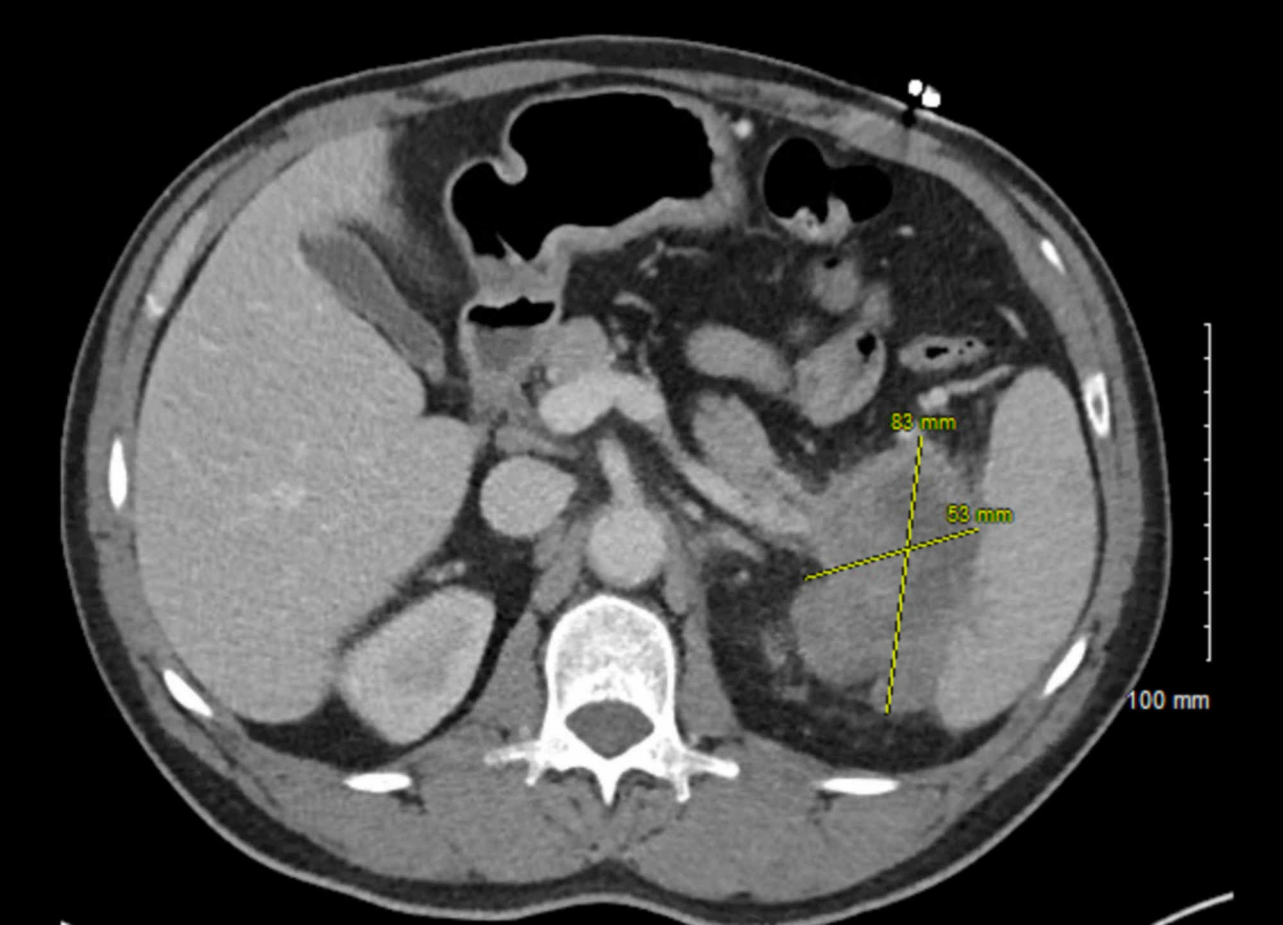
Computed tomography scan of the abdomen showing an 8.3 x 5.3 cm mass centered around the splenic hilum and tail of the pancreas.
